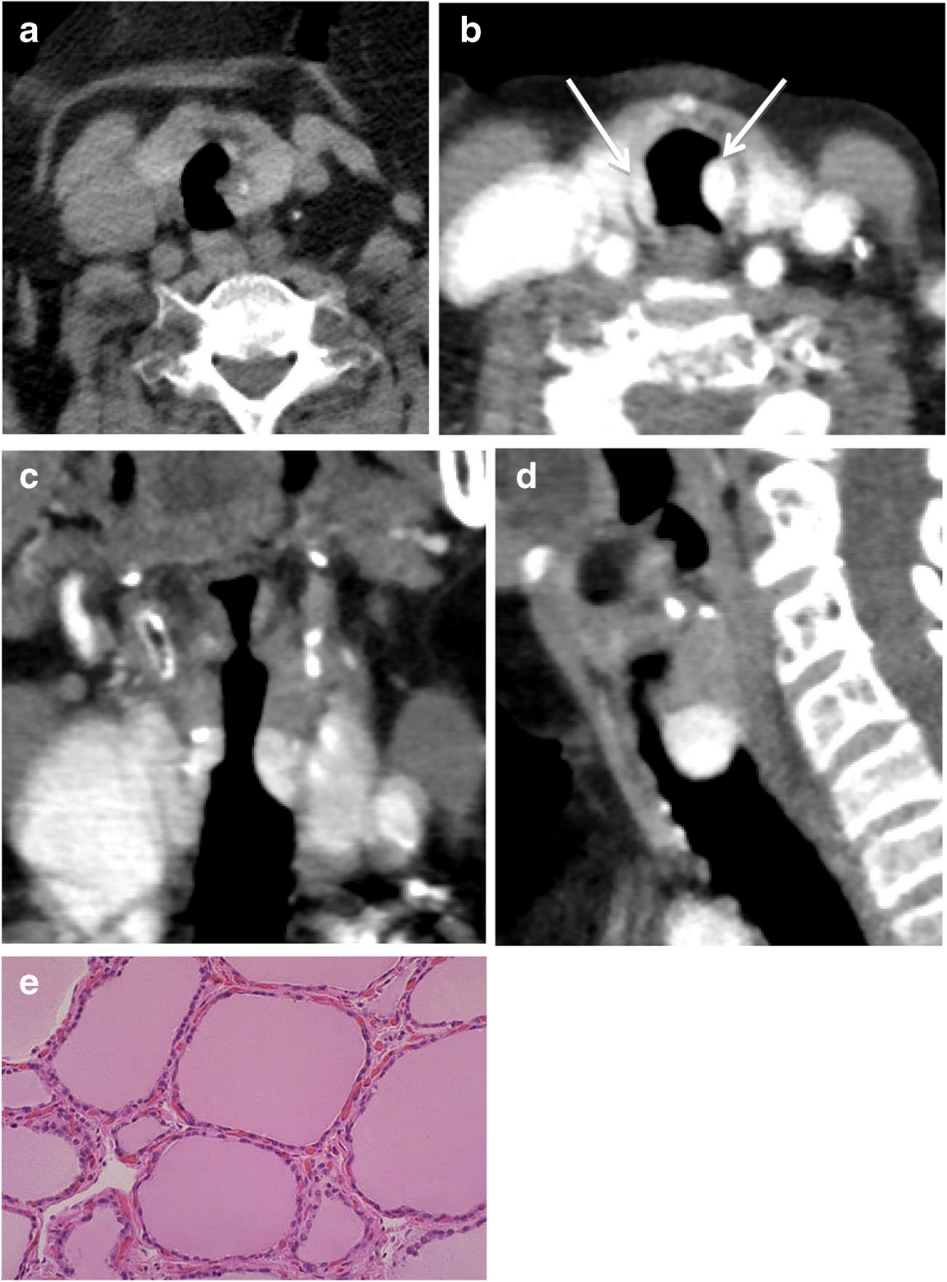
Seventy-nine-year-old woman who presented with stridor. a Axial CT image acquired without intravenous contrast followed by b axial, c coronal, and d sagittal intravenous contrast enhanced imaging. There is avidly enhancing endoluminal tissue that enhances similarly to the thyroid gland at the same level (b, *arrows*). e Pathology of the enhancing soft tissue within the trachea was ectopic thyroid tissue with thyroid follicles of different size
